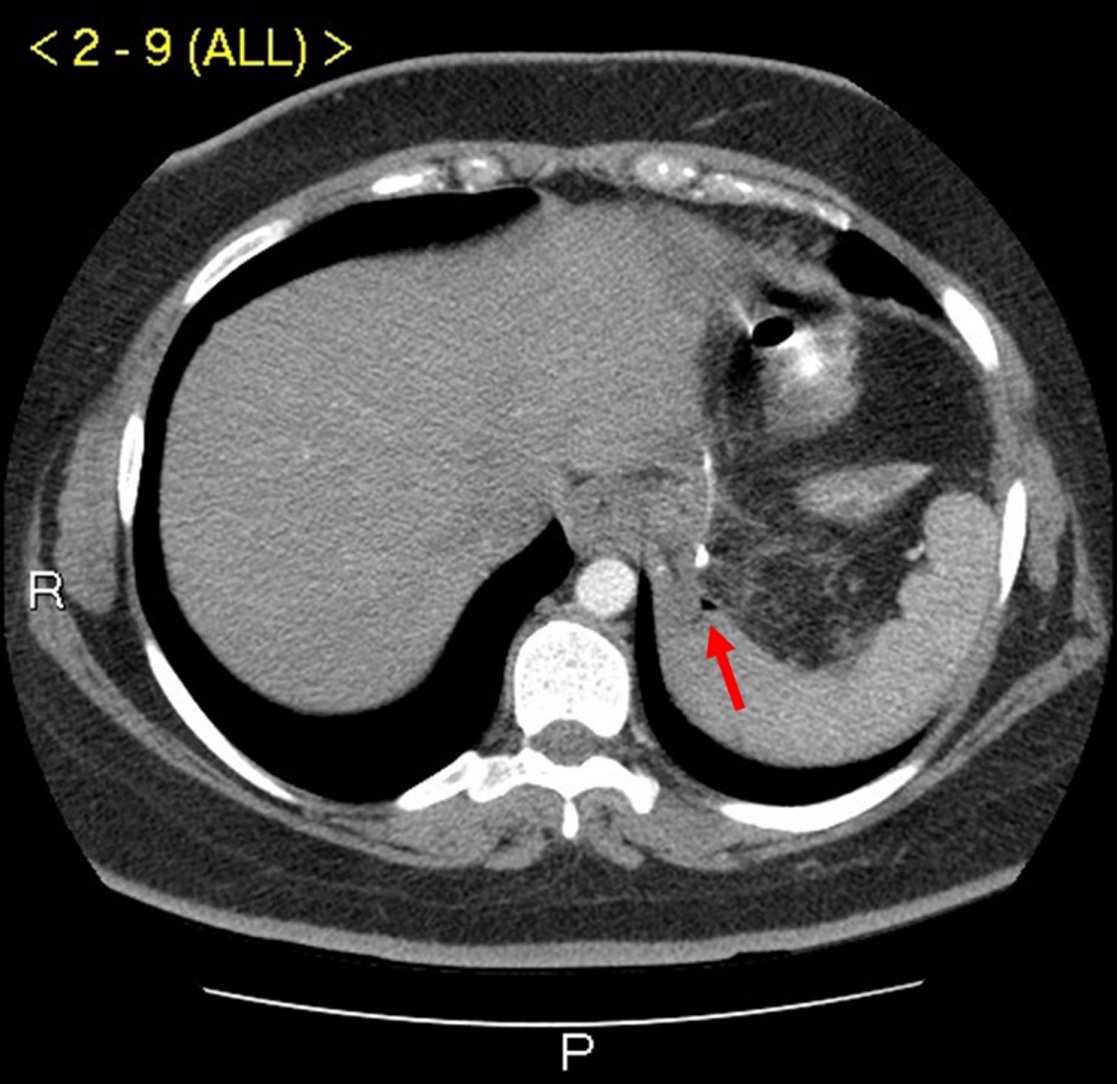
CT scan with intravenous and oral contrast showing air and a faint suggestion of oral contrast outside of the suture, late phase (arrow). P, posterior; R, right.
